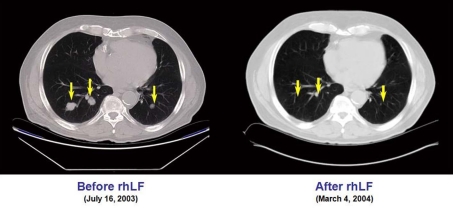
CT scans showing the reduction in size of the pulmonary metastases in case 4.
